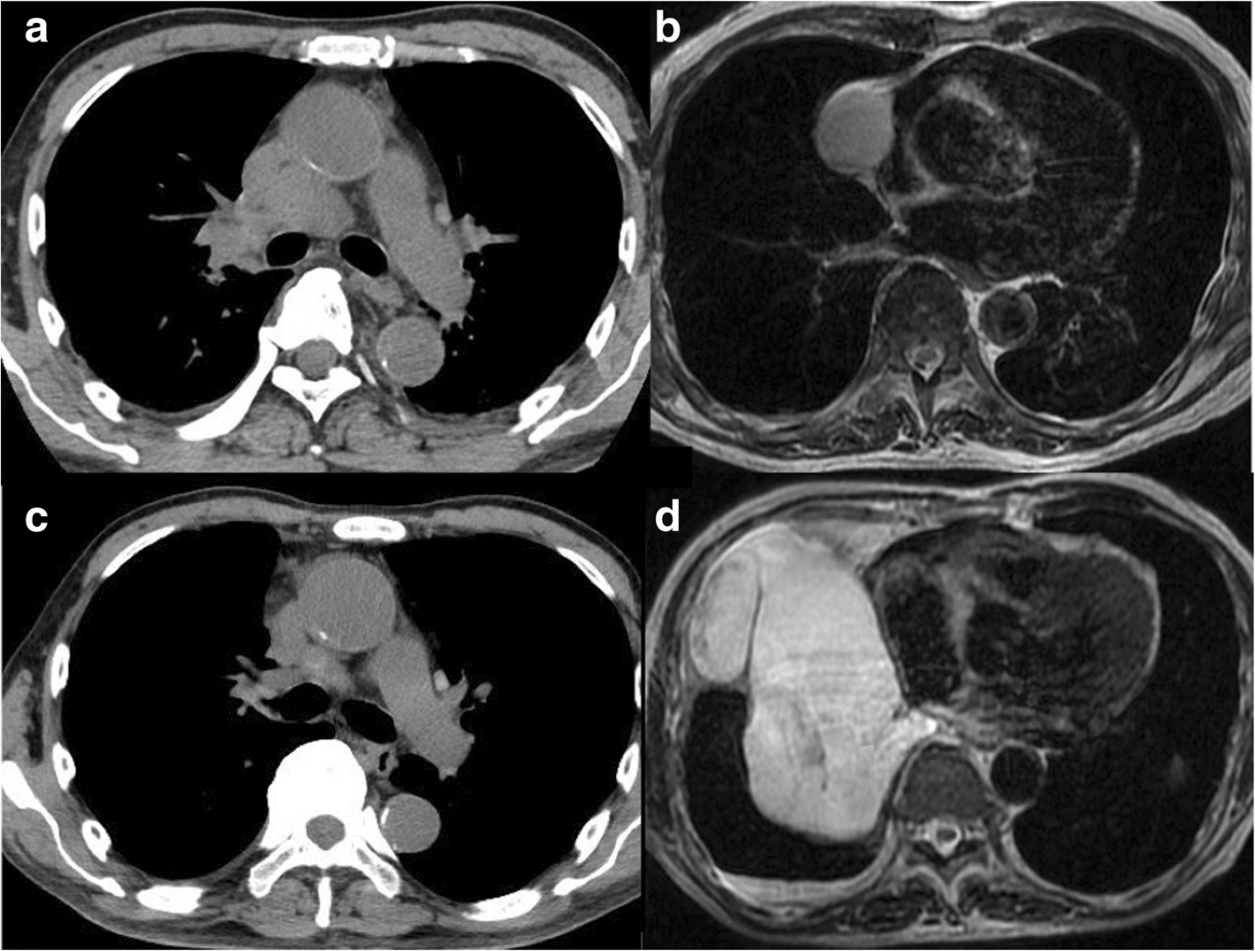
Computed tomography revealed hilar and mediastinal lymph node enlargement (a). Magnetic resonance imaging demonstrated a high-intensity anterior mediastinal tumor on T2-weighted images (b). Treatment with pembrolizumab yielded a partial response from the non-small cell lung cancer (NSCLC) (c) but the mediastinal cyst enlarged extremely rapidly within 3 months (d)
